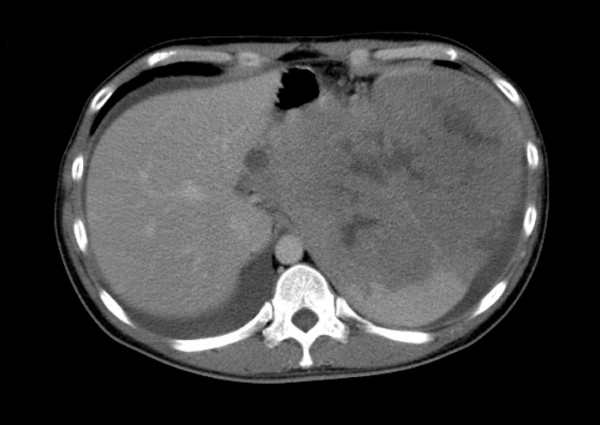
Computed tomography scan of the abdomen showed a large mass over the spleen and adjacent lymph nodes.
